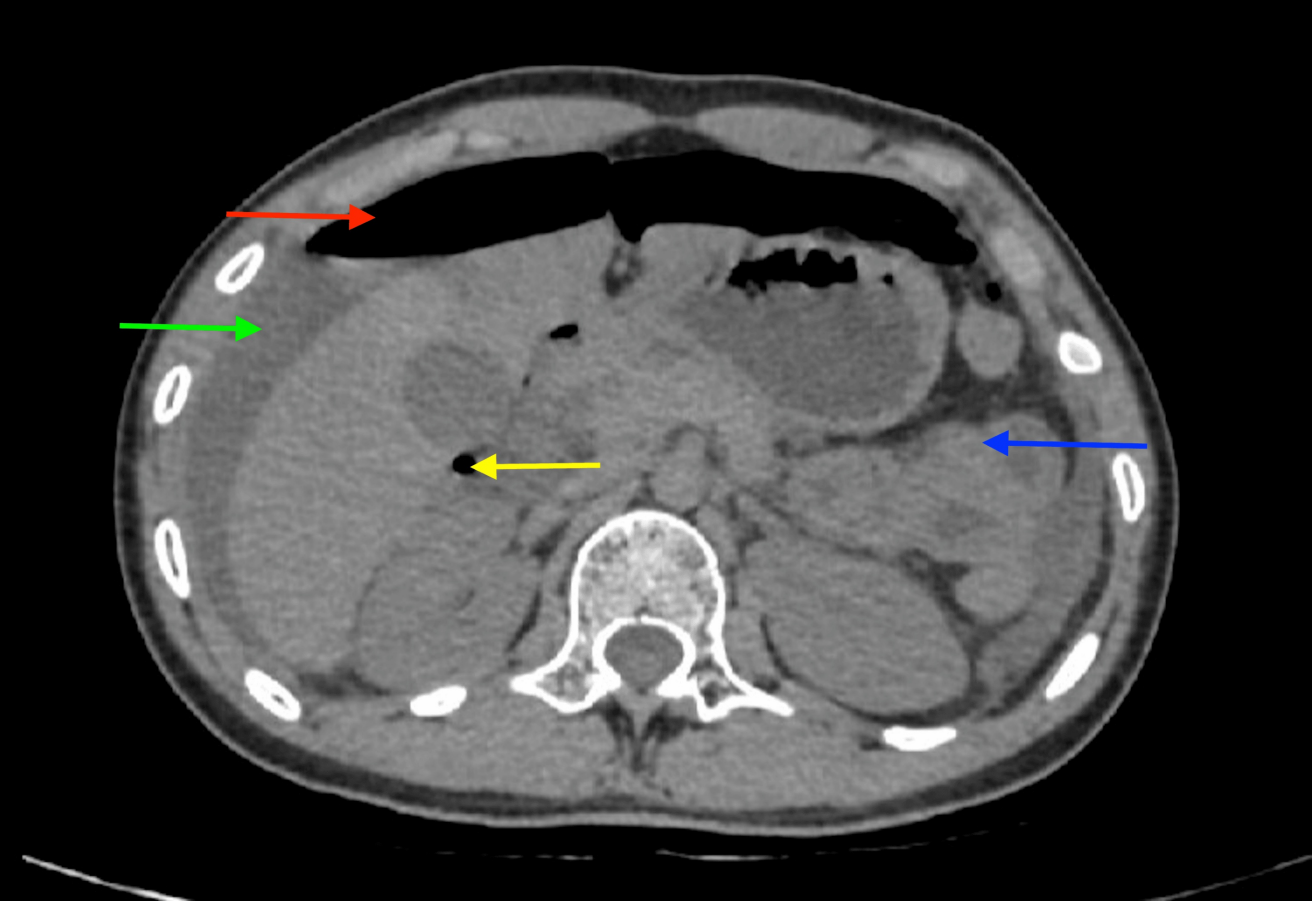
Computed tomography of the abdomen The red arrow shows pneumoperitoneum, yellow arrow shows air pockets, blue arrow shows collapsed ileal loops, and green arrow shows moderate free fluid.
